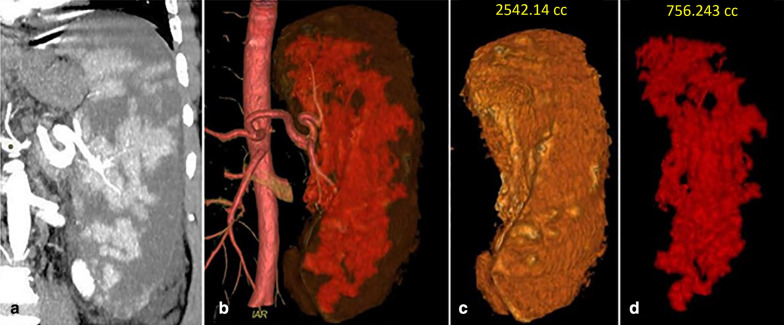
A 63-year-old male underwent PSE as management for cirrhotic hypersplenism. a Coronal reformatted contrast enhanced-CT image at the arterial phase shows large hypoenhancing areas throughout the spleen representing infarctions. b 3D coronal CT angiography image for the aorta and splenic artery shows the infarction of less opacity than viable tissue. c The volumetric analysis of the splenic volume is 2542.14 cc. d The volumetric analysis of the viable tissue is756.3 cc
